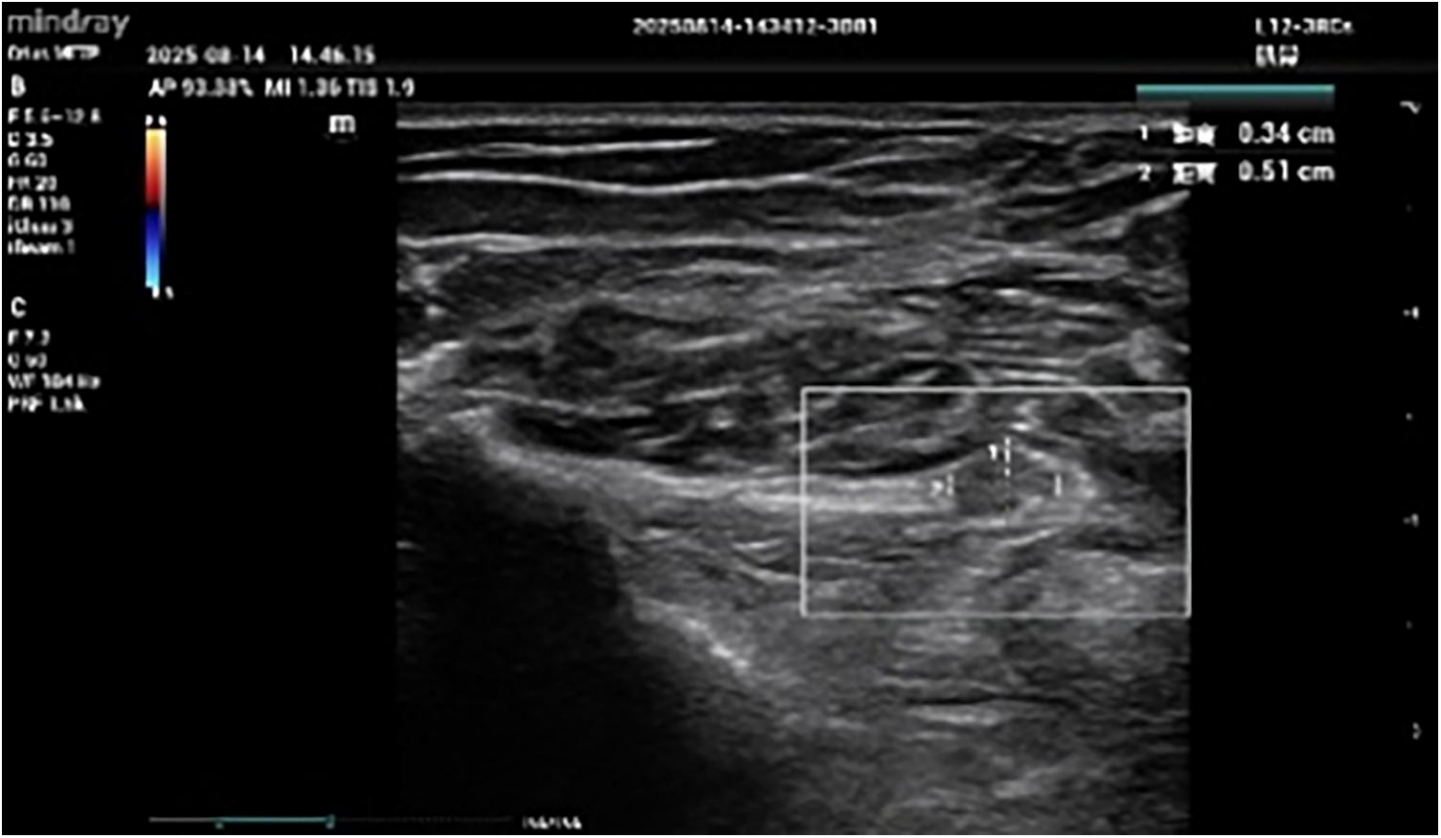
Ultrasound shows the abnormal location of the radial nerve. This figure displays the site of radial nerve thickening in the patient identified via ultrasound, which is one of the radial nerve thickening sites marked in the legend. All subsequent acupuncture treatments for the patient were adjusted based on the thickened nerve sites detected by ultrasound.
